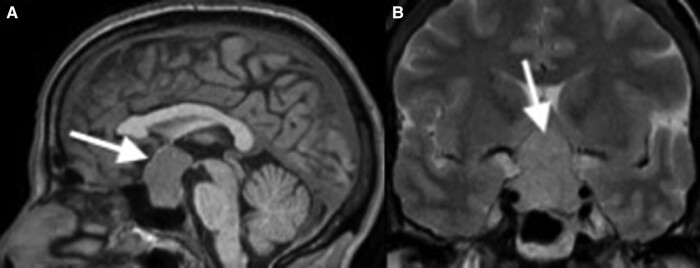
Initial magnetic resonance imaging scan of the pituitary showing a large 2.1 × 2.5 × 3.3-cm macroadenoma with invasion into the right cavernous sinus and with upward displacement and flattening of the optic chiasm. A, Sagittal view. B, Coronal view.
